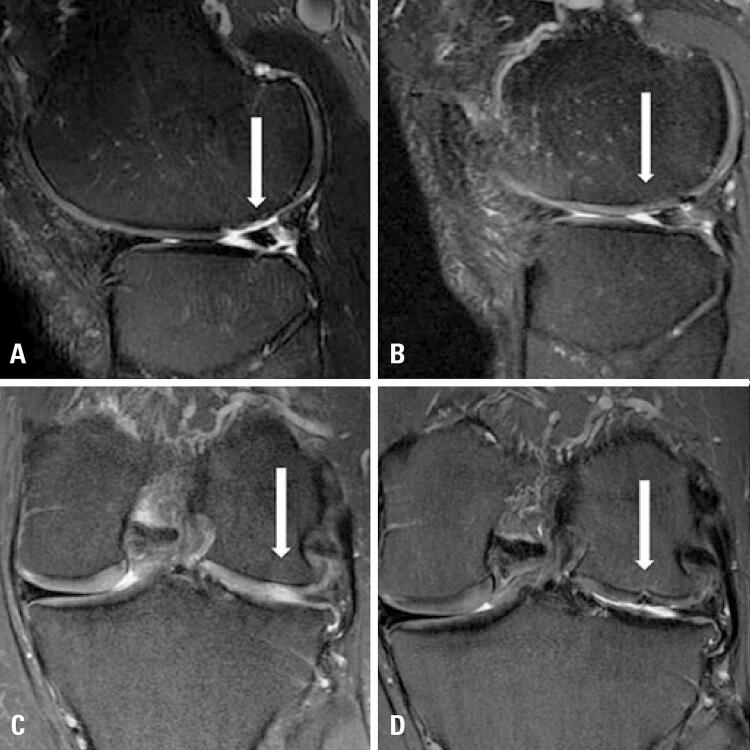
Magnetic resonance imaging of the lesions before and after 12 months of treatment. (A) Sagittal T2-weighted section before surgery, showing the focal chondral lesion (arrow); (B) Same section 12 months after chondrocyte implantation, demonstrating satisfactory filling of the lesion with tissue similar to hyaline cartilage around the lesion (arrow); (C) Same examination of figure 5A, but in frontal section; (D) Same examination of figure 5B, but in frontal section
